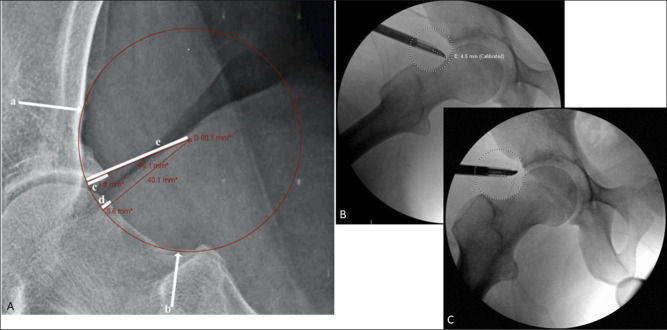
A, Image demonstrating the preoperative template for a patient on a Dunn lateral radiograph using the femoroacetabular impingement resection arc technique. The best fit circle is used to lay tangent to the anterior inferior iliac spine (indicated with the “a” arrow) and the superolateral femoral neck base (indicated with the “b” arrow). Resection depth is measured on the acetabular side (C) and the femoral side (D) and measured as shown in the figure. B, The intraoperative recreation of a preoperative plan on a separate patient showing area of intended resection within the arc of the circle. C, Post resection Dunn lateral radiograph showing the patient demonstrated in (B). Reproduced from Matache et al 2021 with permission from Journal of Arthroscopic Techniques.
